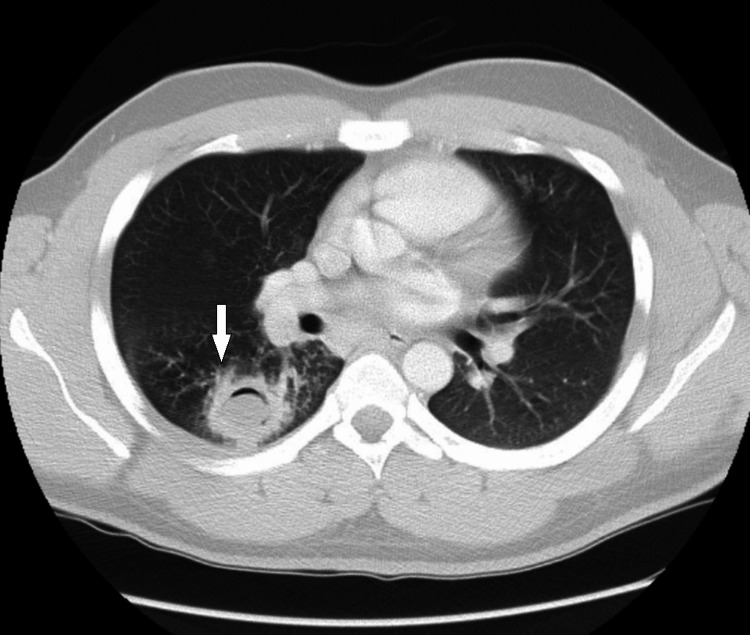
Chest CT scan showing the right lung abscess (arrow)
